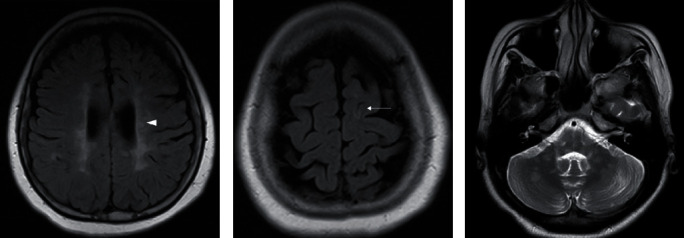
MRI of two different MS patients in our center: (a, b) a 30-year-old female with SPMS, disease duration of 10 years, showing periventricular (arrowhead) and juxtacortical (arrow) lesions on axial FLAIR images and (c) 27-year-old female with RRMS, disease duration of 3 years, showing infratentorial lesions on axial T2WI.
